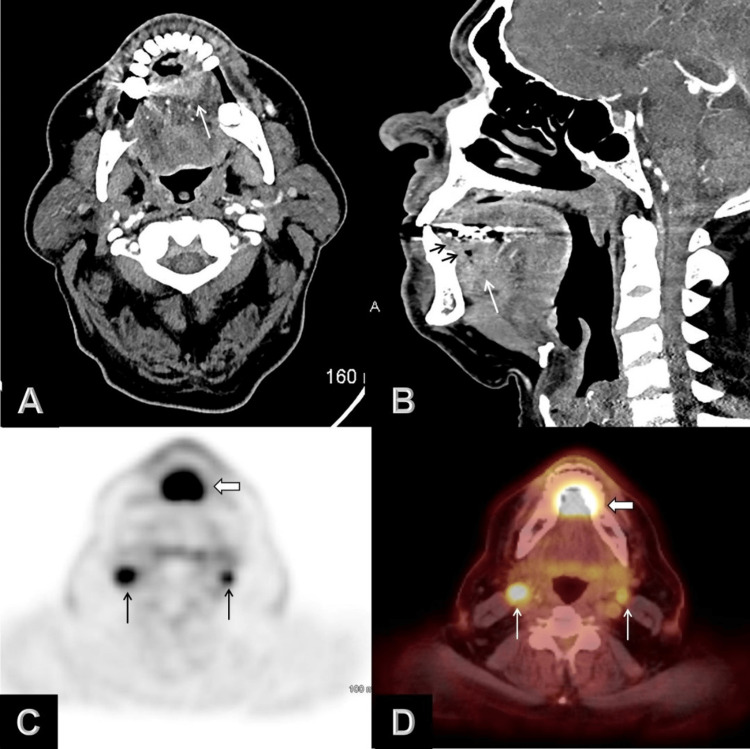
Axial (A) and sagittal (B) contrast-enhanced CT images show an ulcerative mass (white arrows) involving the floor of the mouth and anterior midline ventral aspect of the tongue with infiltration of the genioglossus muscle. The mass infiltrates the gingival mucosal lining of the lingual mandibular cortex (black arrows). Axial 18F-FDG PET (C), and axial fused PET/CT (D) images demonstrate the floor of the mouth mass with avid FDG uptake (wide arrows) and bilateral level 2 FDG-avid lymph nodes (arrows). CT: computed tomography; PET: positron emission tomography; FDG: fludeoxyglucose
